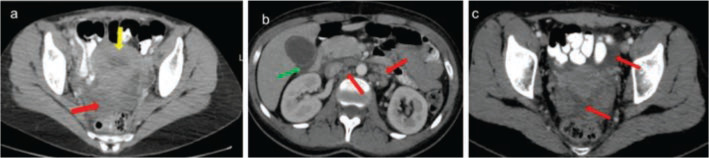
Abdominal CT images of patients 1 and 2. (a) Patient 1, axial CECT image: Fluid collection at the lower pelvis (red arrow) and mild fluid collection in the uterine cavity (yellow arrow). (b) Patient 2, axial CECT image: Pericholecystic fluid collection (green arrow); enlarged lymph nodes (red arrows). (c) Patient 2, axial CECT image: Fluid collection in the lower pelvis.
